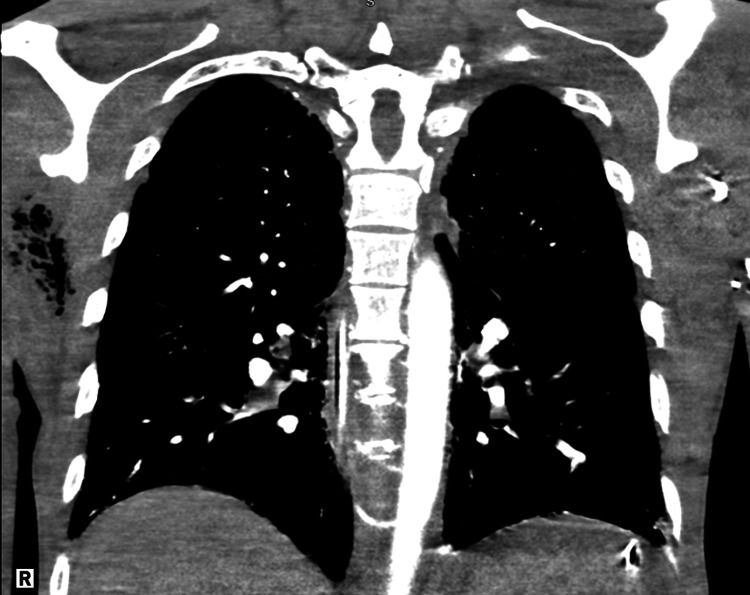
CT angiography chest with extensive soft tissue gas and edema in the right shoulder and axilla
